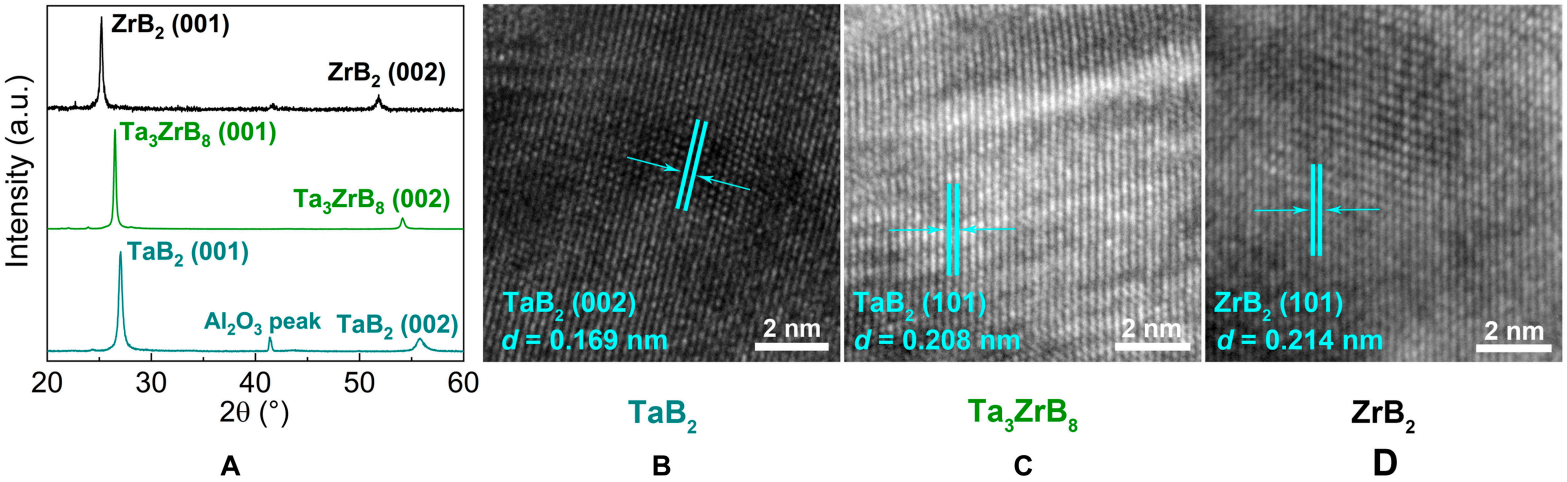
(A) The measured x-ray *θ*/2*θ* scan patterns. (B to D) HRTEM images of the as-deposited TaB_2_, Ta_3_ZrB_8_, and ZrB_2_ films, with selected lattice spacings indicated. a.u., arbitrary units.
